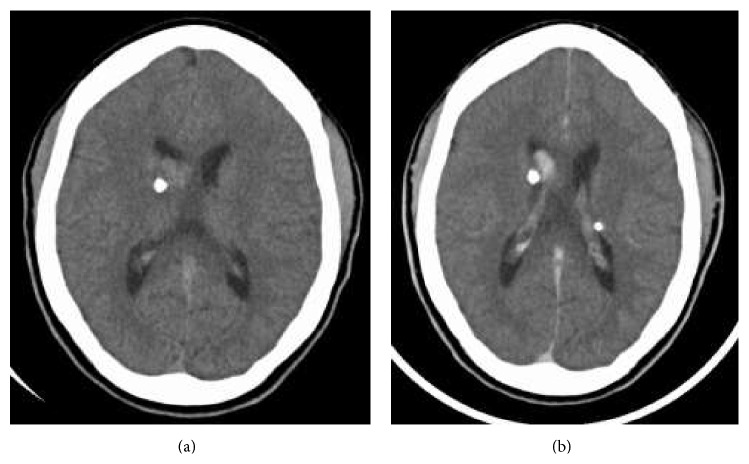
Axial noncontrast CT demonstrating a 12 × 18 mm partially calcified, enhancing soft tissue mass in the anterior body of the right lateral ventricle (a). Axial CT with contrast showing SEGA enhancement but no enhancement of tubers (b).
